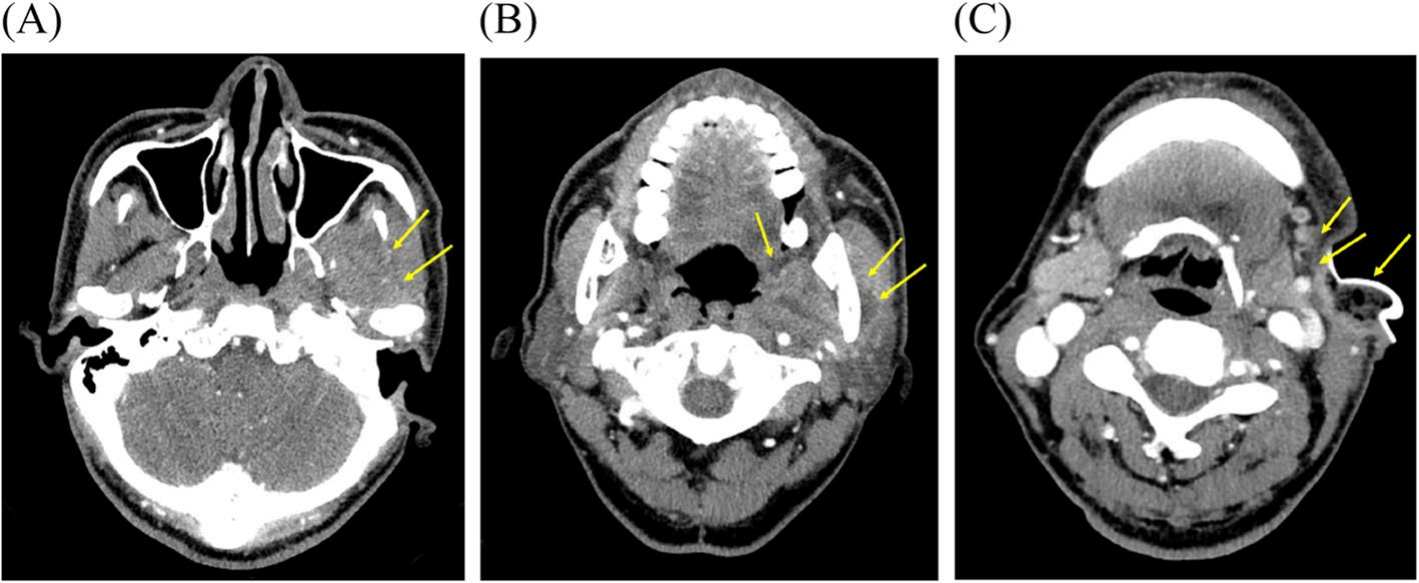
Neck angiography CT images 1 month after the first visit (case 2). Heterogeneous lesions (yellow arrows) were decreased but remained. A Masseteric space. B Parapharyngeal and masseteric space. C Submandibular space
